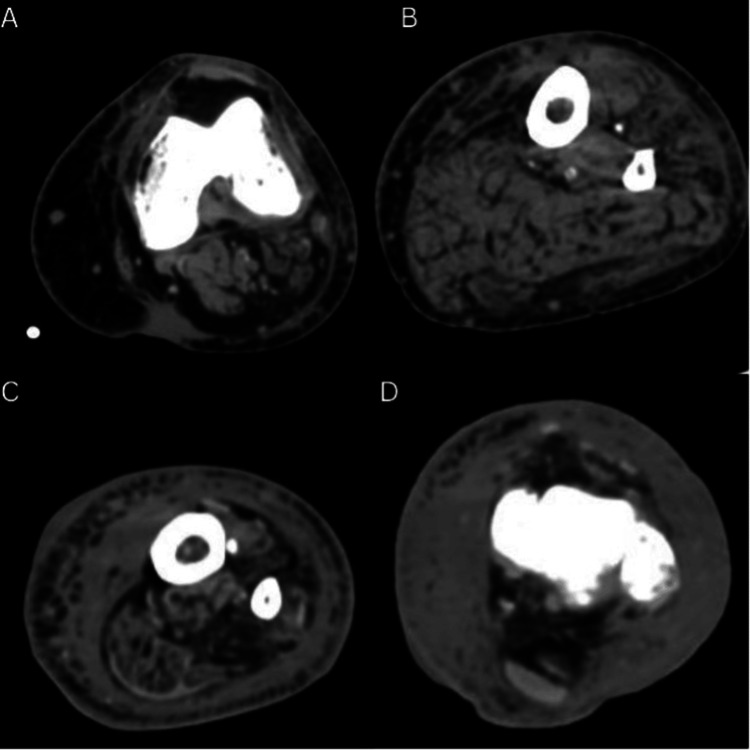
CT scan of the left leg at different levels (A – D) Severe oedema at the subcutaneous level involved all of the leg (at the knee level (A); upper third of the leg (B); lower third of the leg (C)). The muscular plane is shown without any alterations. The ankle region (D) features more prominent oedema.
